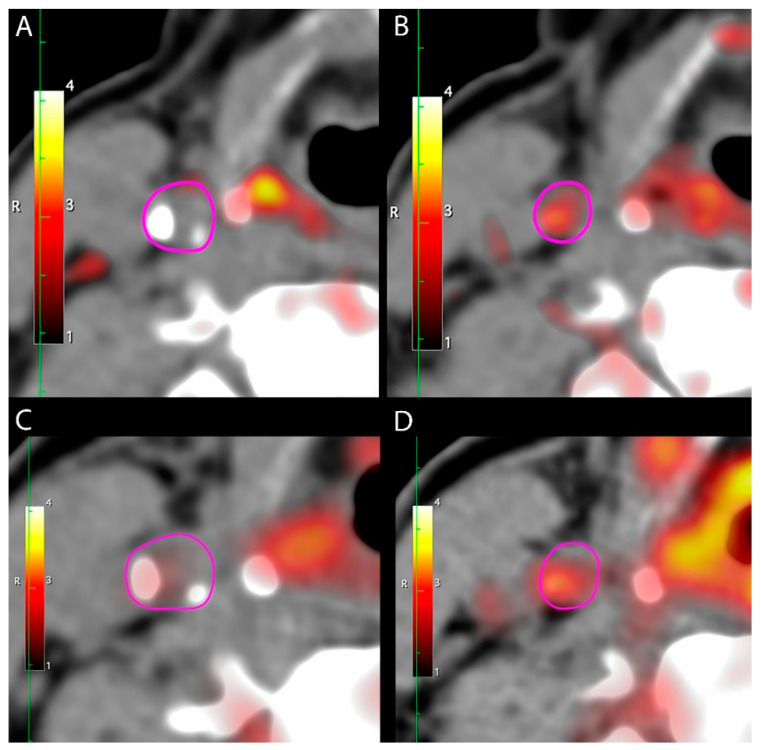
PET/CT images at baseline with high and low uptake of [^64^Cu]DOTATATE. Representative baseline axial images showing the carotid artery outlined in pink. (A). An axial slice with calcifications and low uptake of [^64^Cu]DOTATATE (SUV_mean_ = 0.9). (B) An axial slice with minimal calcifications and high uptake of [^64^Cu]DOTATATE (SUV_mean_ = 1.4). (C,D) [^18^F]FDG uptake in the same locations for comparison.
